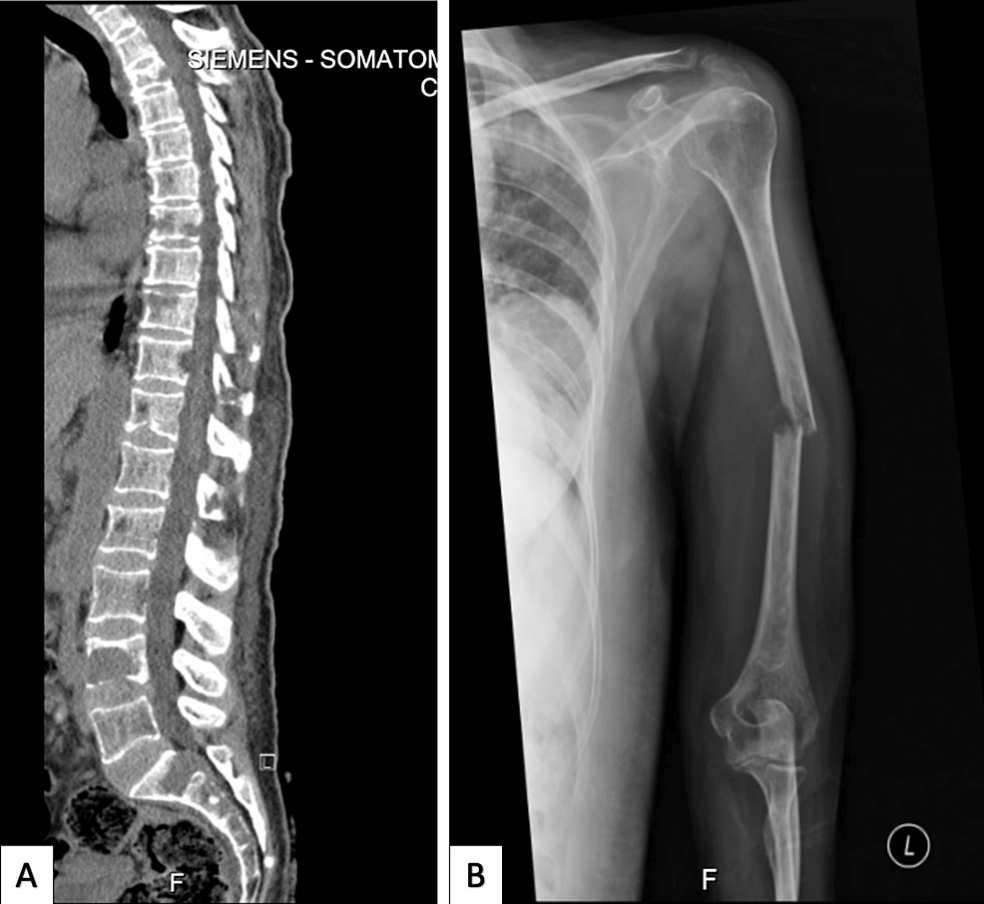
(A) CT of the whole spine shows extensive metastatic disease involving the spine. (B) The left upper limb X-ray shows a humeral shaft transverse fracture.
